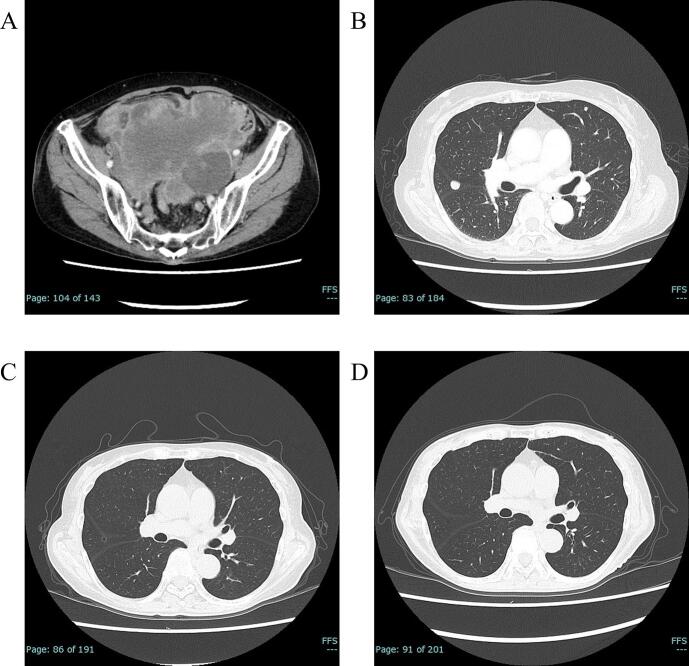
Contrast-enhanced computed tomography findings. (A) Heterogeneously enhancing irregular pelvic mass. (B) Multiple pulmonary nodules consistent with metastatic disease. (C) Partial response achieved after chemotherapy. (D) Sustained complete response during maintenance therapy.
